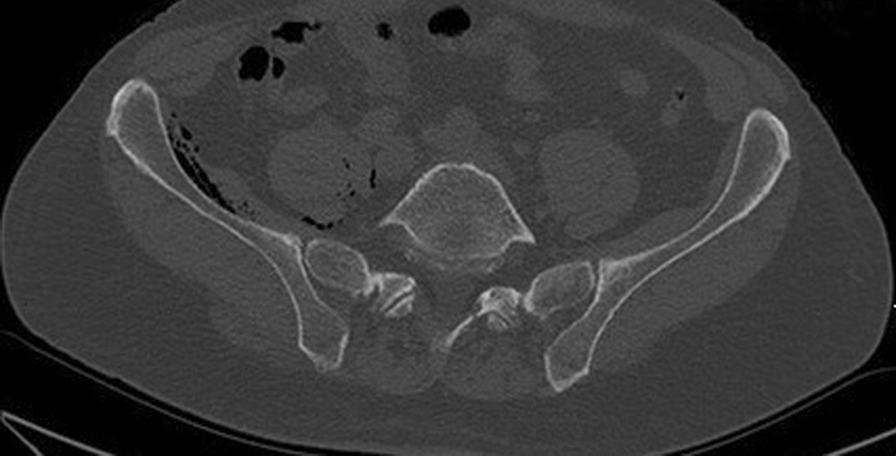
An axial slice of a CT showed the gas flap between ilium and the iliopsoas muscle in the right hip
